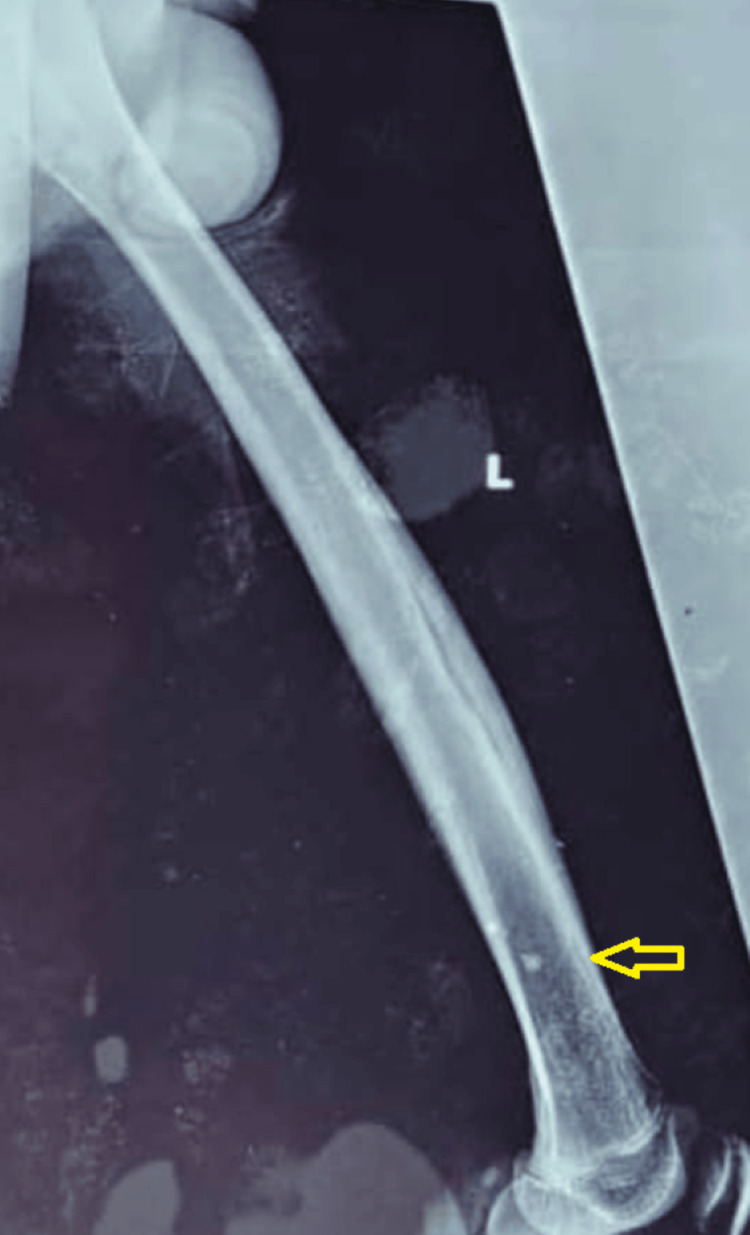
Plain radiography of the left femur (anteroposterior view) The radiograph demonstrates marked cortical thickening and sclerosis localized to the mid-to-distal diaphysis (yellow arrows). An elongated, radiolucent intracortical track is visible, representing a cloaca or chronic tract, alongside irregular medullary density. These features are characteristic of subacute to chronic osteomyelitis, with no evidence of acute pathological fracture or knee joint involvement.
